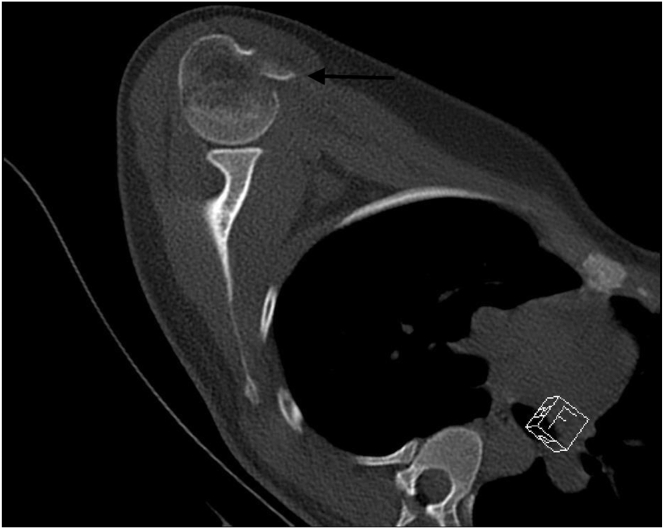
Axial computed tomography image showing a clearly displaced avulsion fragment of the lesser tuberosity (arrow). (R, right shoulder).
